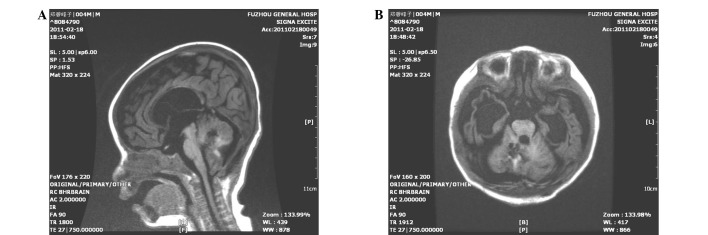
Post-operative review showing the disappearance of the tumor and the subsidance of the hydrocephalus. (A) Sagittal and (B) axial magnetic resonance imaging (MRI).
